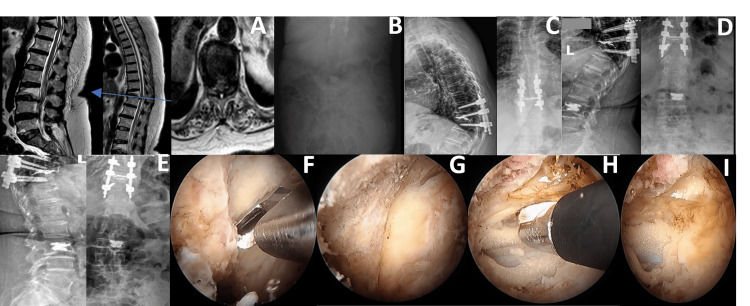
Thoracolumbar decompression and stabilization in Case 3. (A) Axial and sagittal T2-weighted MRI showing a pathological fracture at T10 with cord compression and another pathological fracture at L3. (B) AP abdominal X-ray taken on postoperative day 3, showing signs of ileus. (C) AP and lateral spine X-rays taken nine months postoperatively showed screws in good position and successful kyphoplasty after the patient underwent endoscopic decompression of D10 using a biportal endoscopic technique, ligamentotaxis, D10 kyphoplasty, and transpedicular screw fixation at D9, D10, and D11 bilaterally, and L3 kyphoplasty. (D) AP and lateral spine X-rays taken five months postoperatively showed screws in good position and successful kyphoplasty following the same procedure. (E) AP and lateral spine X-rays were taken on postoperative day 2, showing screws in good position and successful kyphoplasty after the same surgical intervention. (F) Intraoperative image showing a unilateral D10 laminectomy. (G) Intraoperative image showing the D10-D11 interlaminar space during the same procedure. (H) Intraoperative image showing contralateral decompression during the same surgical intervention. (I) An intraoperative image shows excellent cord decompression following the procedure AP: anteroposterior
